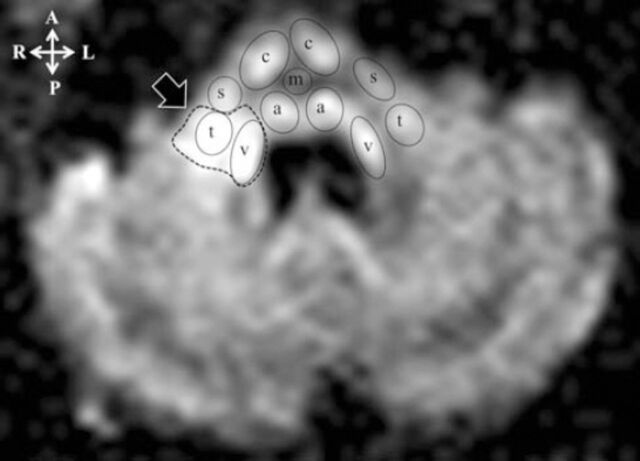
Diffusion weighted MRI (repetition time/echo time/excitation: 9,212/144/1) demonstrated a hyperintense area in the right-sided upper medulla (filled arrow). The hyperintense area (dotted line circle) involved the right vestibular nucleus (v) and spinal tract of the trigeminal nerve (t). c - corticospinal tract, m - medial lemniscus, a - abducens nucleus, s - anterior spinocerebellar tract.^6^
